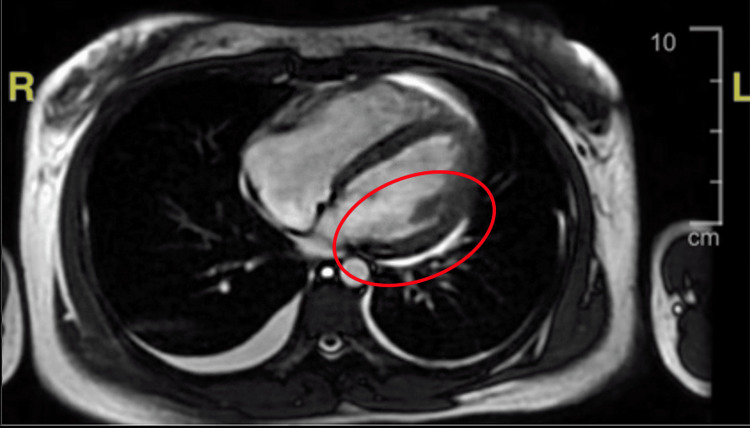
Cardiac MRI (transverse section) Inflammation can be seen as white patches (red circle) within the myocardium after contrast injection. Eccentric dilation of ventricles can be appreciated
